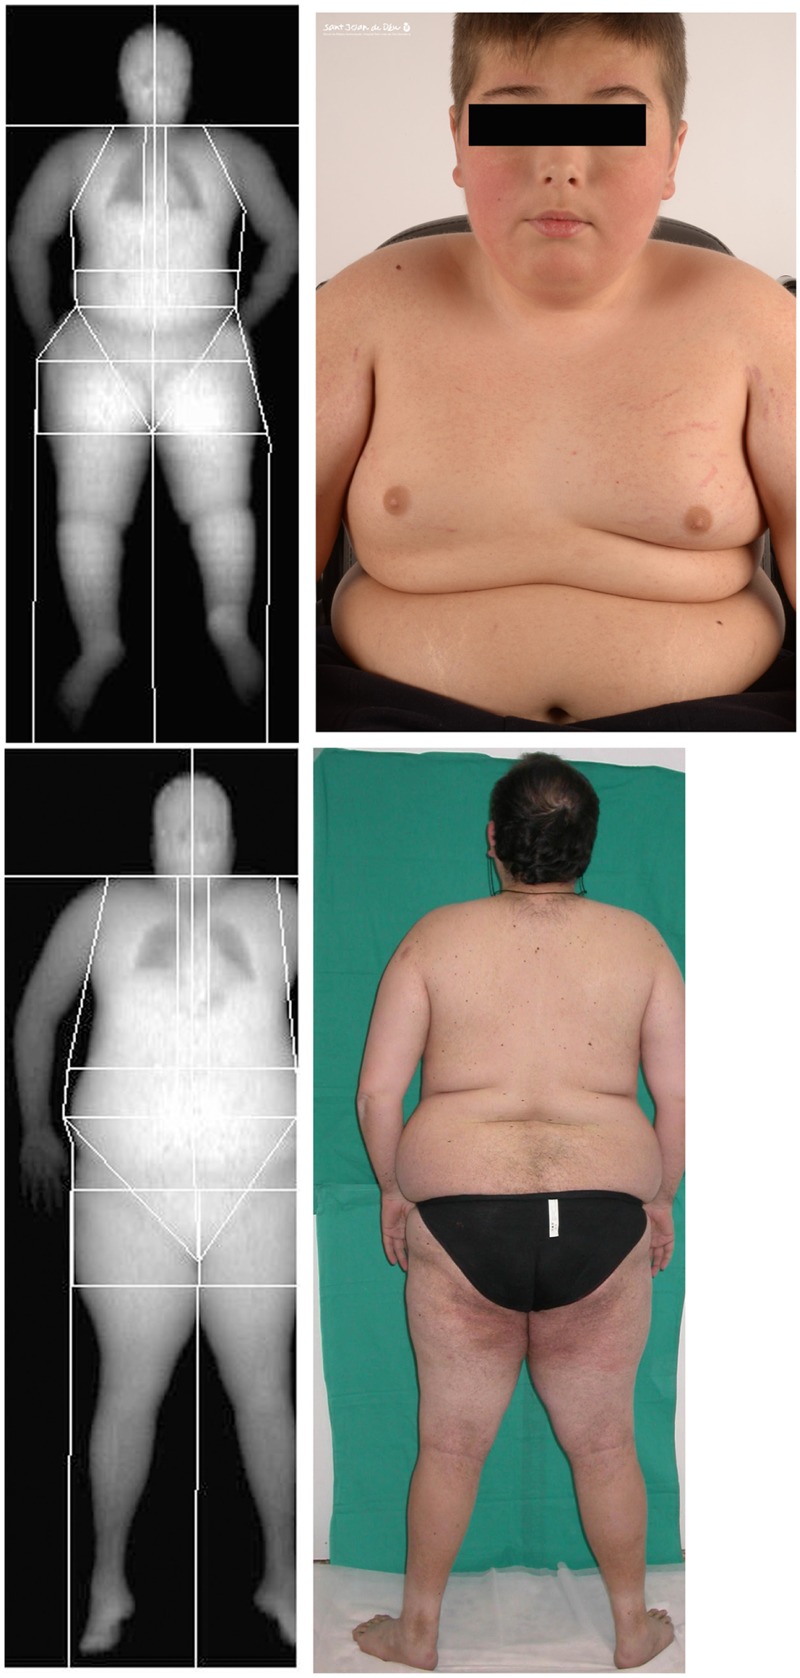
Representative dual-energy X-ray absorptiometry (DXA) scan showing the soft tissues in the different regions analyzed for one of the children with Ullrich Congenital Muscular Dystrophy (UCMD), Top, and an adult patient with Bethlem Myopathy (BM), Bottom, and their corresponding clinical images. Written informed consent was obtained for the publication of this image.
